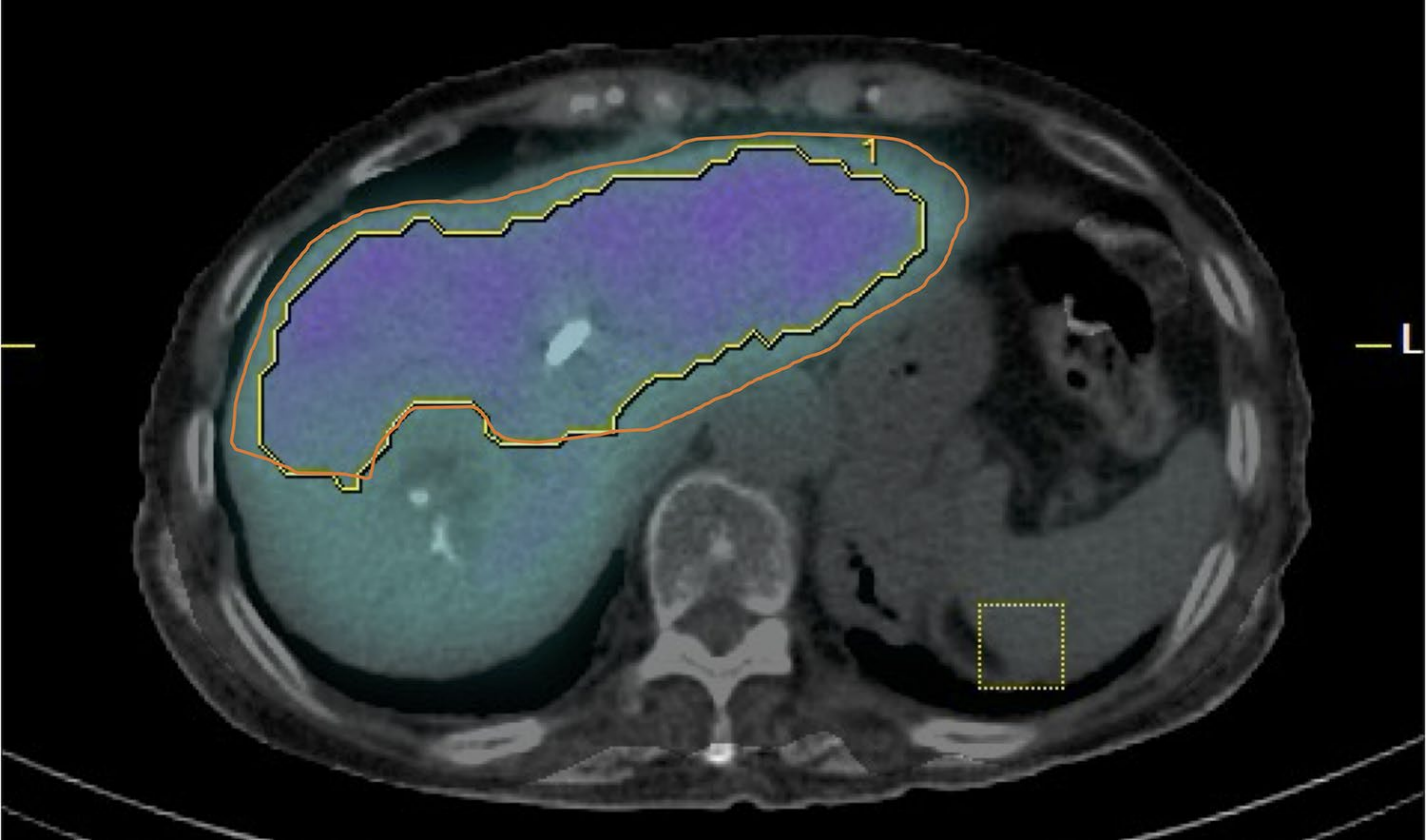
Difference between single-photon emission computed tomography (SPECT) and CT contours in case of right hemihepatectomy after portal vein embolization for right branch of portal vein. In this case, liver accumulation was low, and SPECT contour of remnant liver (yellow line) was located inside CT contour (orange line)
